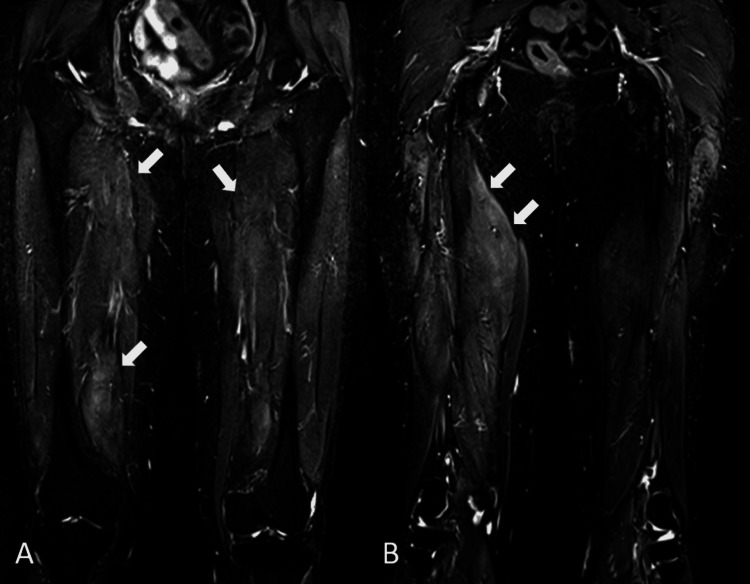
MRI of the thighs Coronal T2 TIRM sequence images through the anterior (A) and posterior (B) thighs show hyperintense signals (indicated by arrows) in the thigh muscles on both sides consistent with myositis MRI: magnetic resonance imaging; TIRM: turbo inversion recovery magnitude
